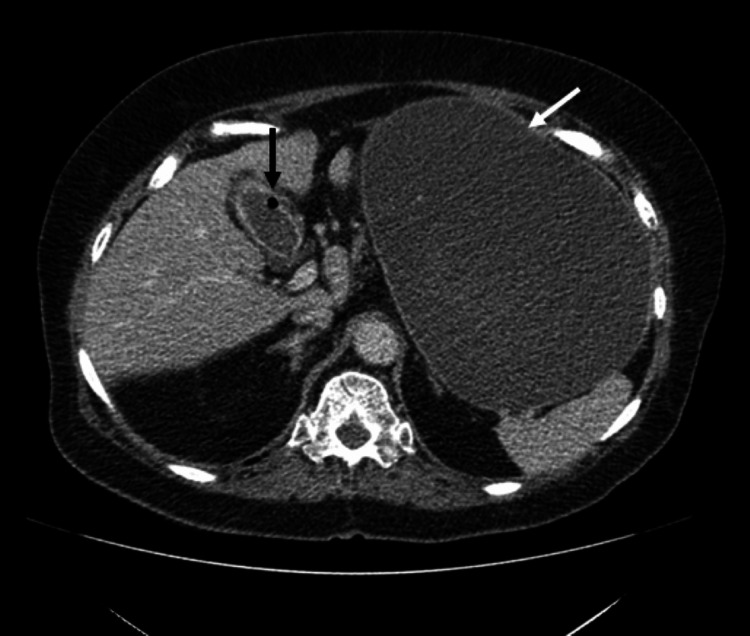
Axial portal venous CT shows speck of gas in the gallbladder in keeping with a fistula (black arrow). Massive gastric distension again noted (white arrow).
